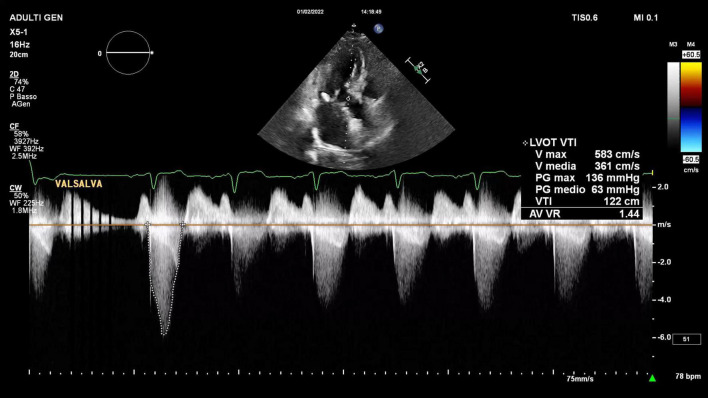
Two-dimensional trans-thoracic echocardiogram continuous wave Doppler representation of LVOT peak gradient at rest and during Valsalva maneuver.
